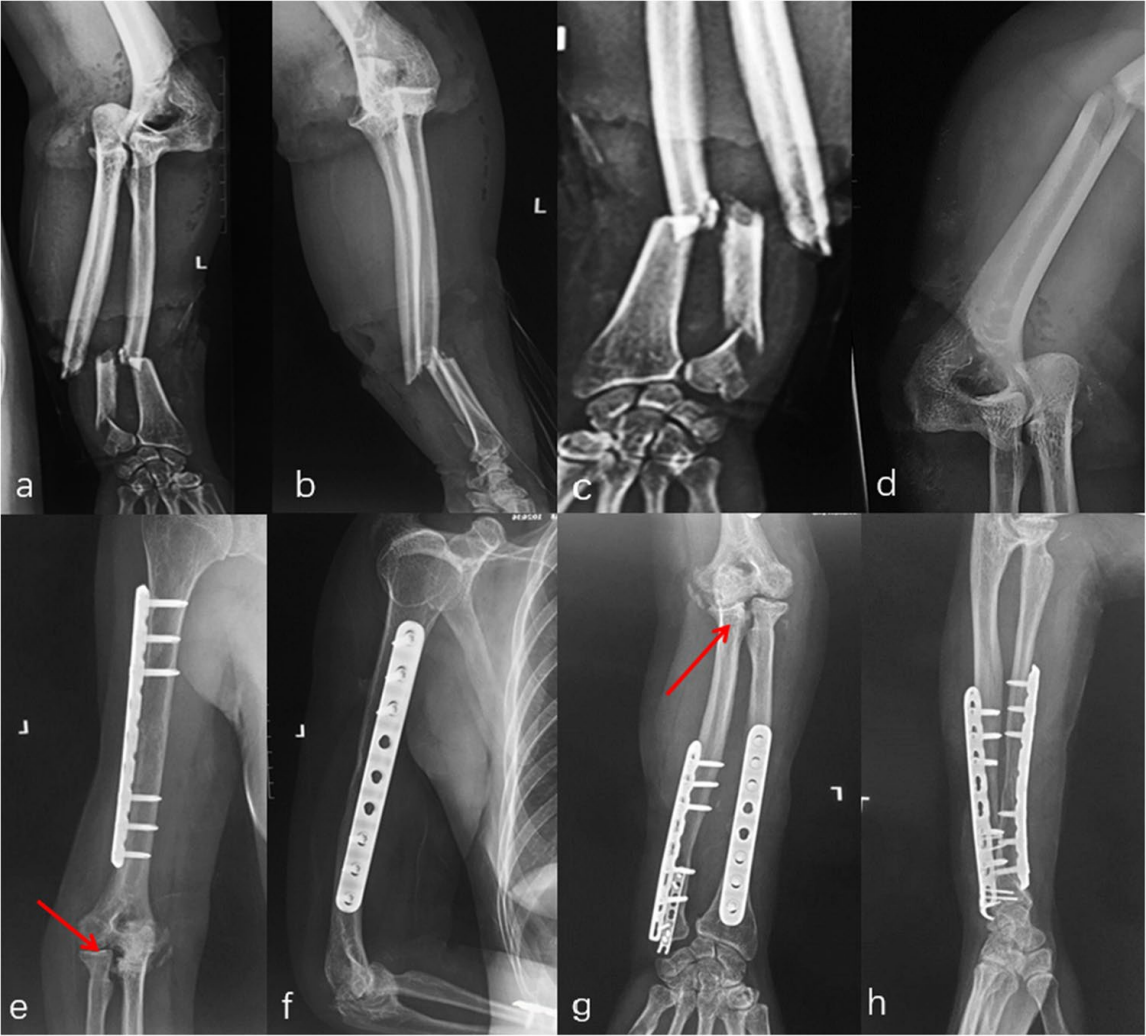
Initial radiographs of the elbow and wrist showed the DDE and a multiple fracture (a–d). All fractures were reduced by internal fixation and subsequent X-ray revealed a grade 1 heterotopic ossification at a 12 monthly follow-up (arrow) (e–h)
